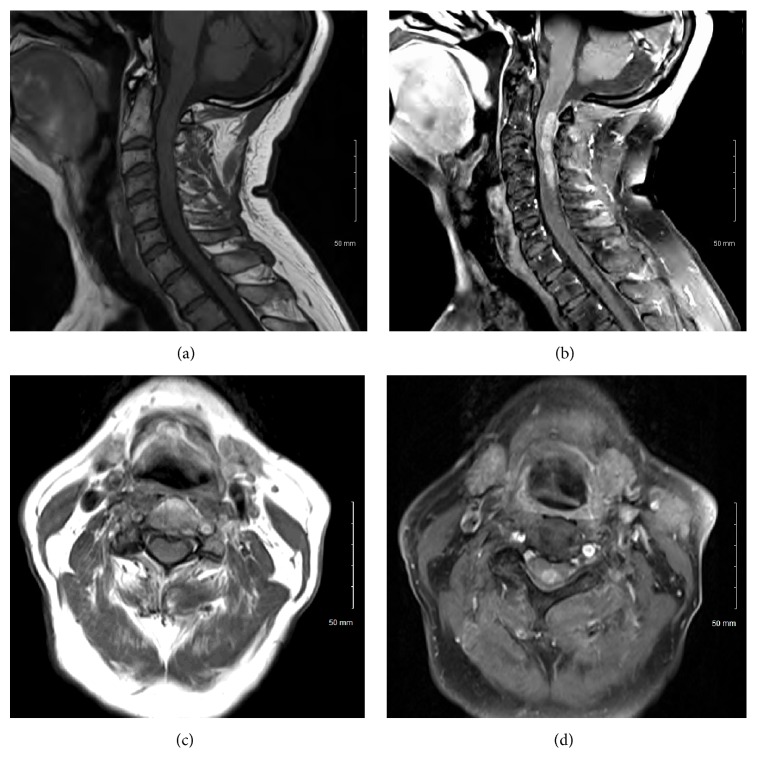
Preoperative T1-sequence MRI of the cervical spine. Sagittal images without (a) and with (b) gadolinium contrast material and axial images without (c) and with (d) gadolinium, showing an eccentric heterogeneously gadolinium-enhancing intramedullary mass between C2 and C4.
